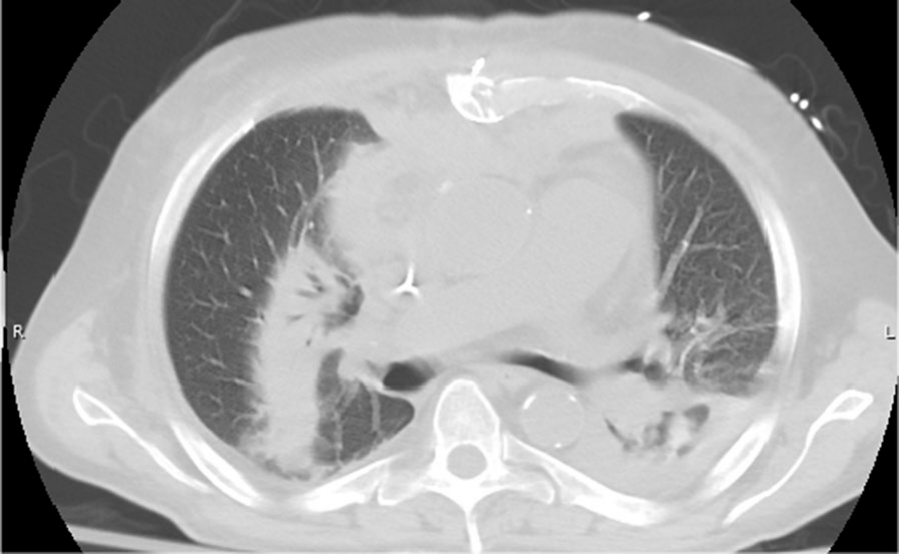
Computed tomography of chest showing bilateral infiltrates and bilateral pleural effusion
